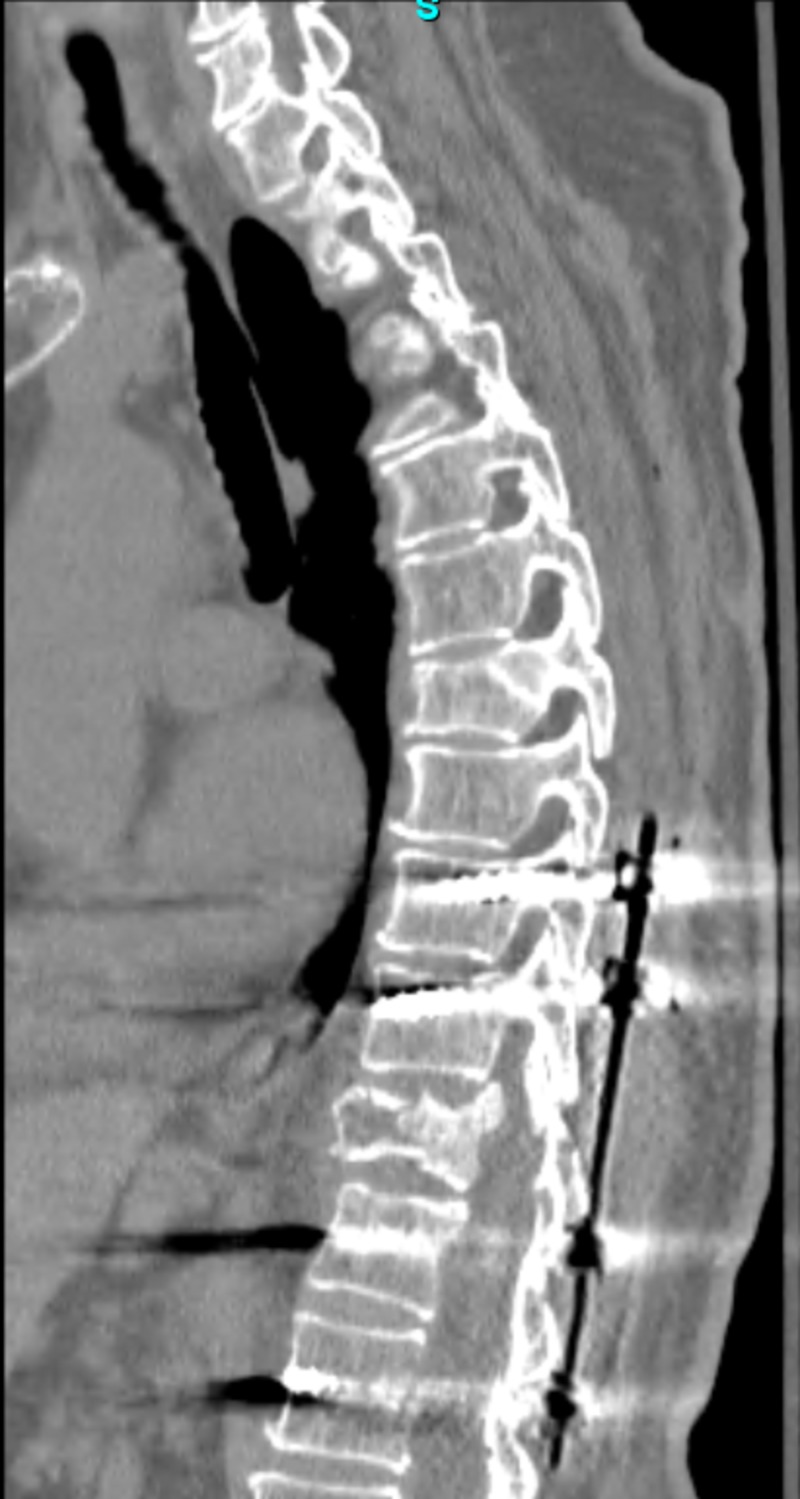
Post-operative computed tomography demonstrating T9-L1 pedicle screw fixation
